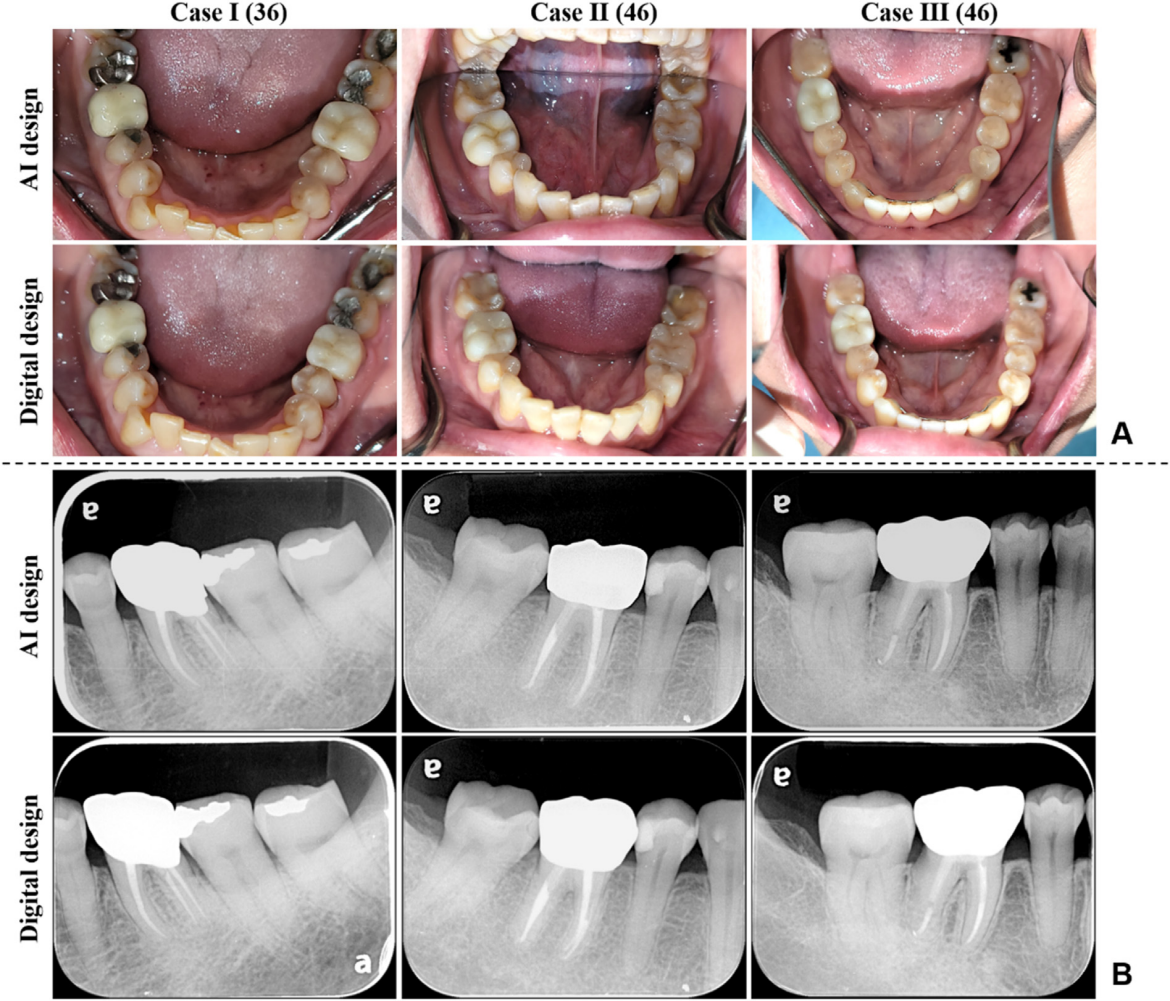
Medical image analysis of prosthetics installed in the oral cavity of subjects in human trials. (A) The optical image of the crown in the mouth is recorded by the camera. (B) Through the dental X-ray record the penetrating images of the crown in the mouth.
